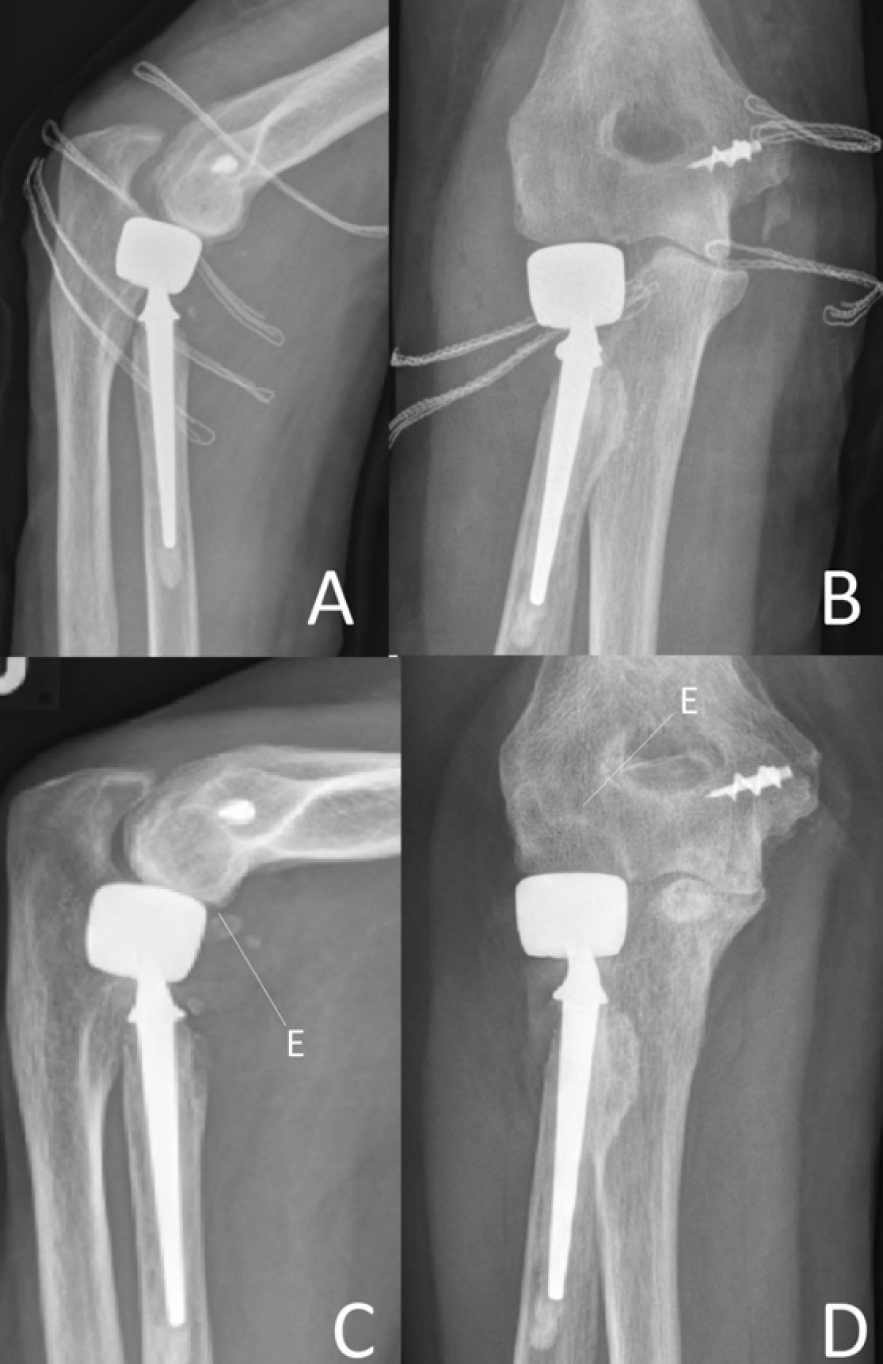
a, b Show post-OP X-rays after bi-polar radial head replacement. Significant overlengthening is visible. It is shown by the head component of the prosthesis exceeding the level of the lateral edge of the coronoid. Also, subluxation is present. The bony structure of the capitulum is intact, but there is no cartilage cover visible anymore. c, d show the same patient 1 year later, where the overlengthening has led to abrasion of the cartilage and some of the subchondral bone. In the lateral image (c), one can see how a spur has developed (e) at the ventral aspect of the capitulum. In the a.p. (d) the abrasion has a half-circle shaped form (e), resulting from flexion motion of the prosthetic head on the capitulum. To this half-circle shaped X-ray finding at the capitulum in the a.p.-view we refer as the “crescent sign”
